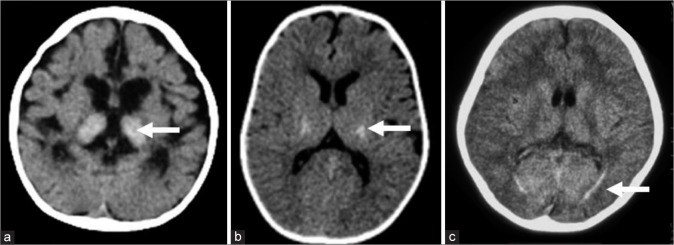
Computed tomography (CT) findings in Krabbe disease. A 9–month- old girl with progressive neuroregression diagnosed as early infantile Krabbe disease - axial CT (a) shows bilateral thalamic hyperdensity (white arrow) with significant cerebral volume loss. Axial CT image in a case with juvenile onset (b) shows bilateral symmetric hyperdensity along posterior limb of internal capsule (white arrow). Axial CT in an adult-onset case (c) depicts hyperdensity (white arrow) along optic radiations bilaterally.
